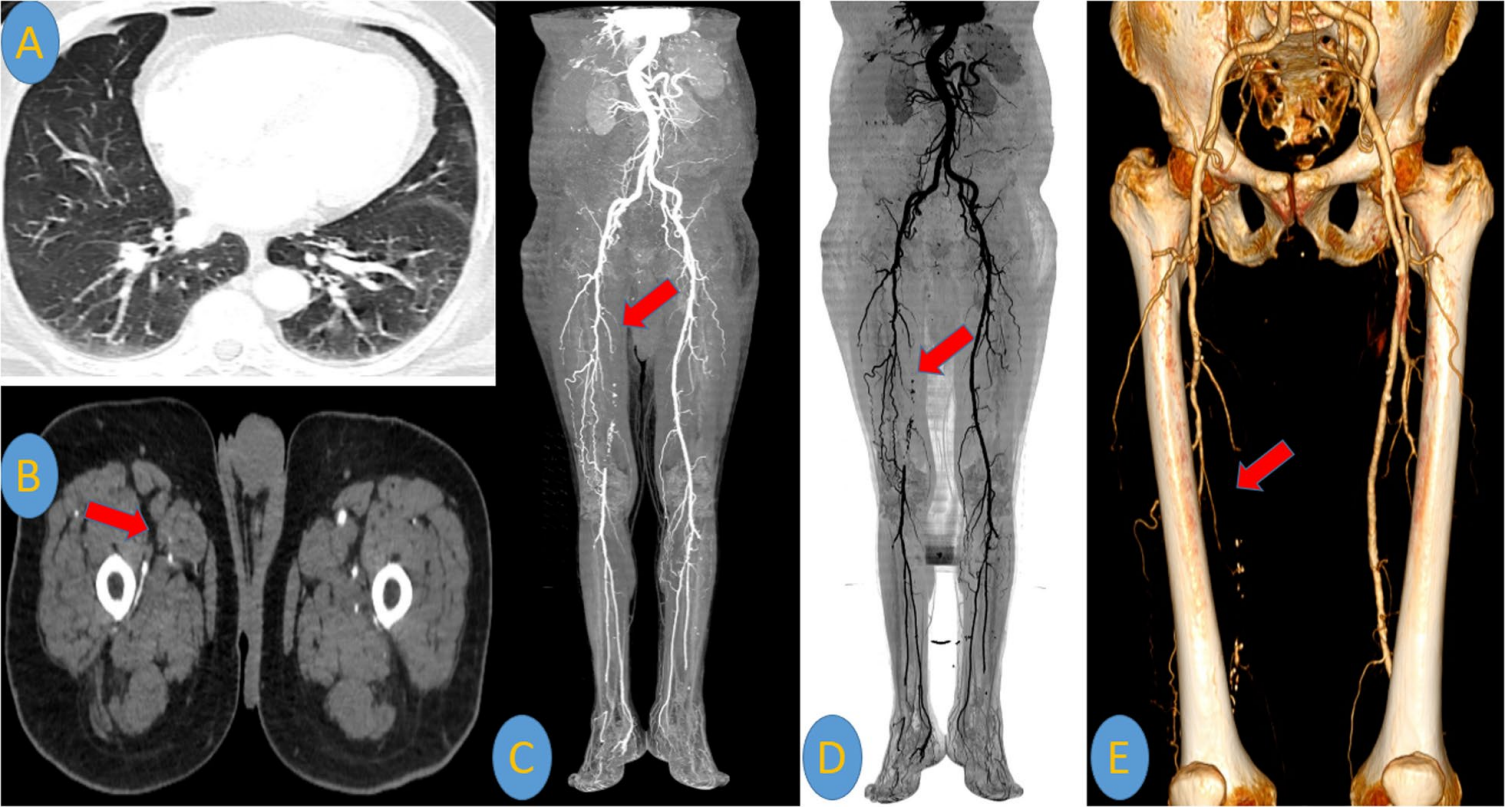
A 67-years-old male patient was proved for COVID-19 infection. He presented to the emergency unit three weeks after infection with severe right leg pain and coldness. [A] Axial image of chest CT revealed faint bilateral sub-pleural COVID-19 pneumonic ground-glass patches. CTA of the lower abdominal aorta and peripheral lower limbs was performed. [B] Axial image of CTA revealed occlusion of the right superficial femoral artery (SFA). [C–E] Volume rendering (VR) reconstruction demonstrated this SFA occlusion. [C] Surface shaded VR image, [D] Negative surface shaded VR image, [E] Colored VR image (red arrows)
